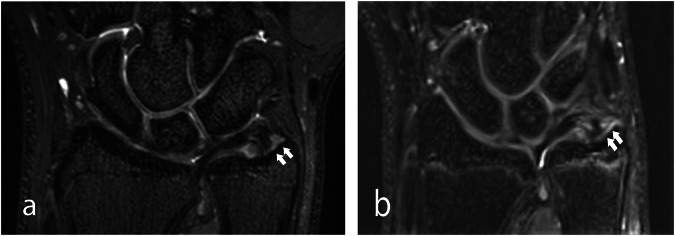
A 19-year-old male with right-sided wrist pain after a sports injury. 3D PD-weighted MRI showed a clearly delineated TFCC injury at the ulnar styloid (arrows) on coronal reformations at both (a) 7 T and (b) 3 T. TFCC, triangular fibrocartilage complex; T, Tesla
